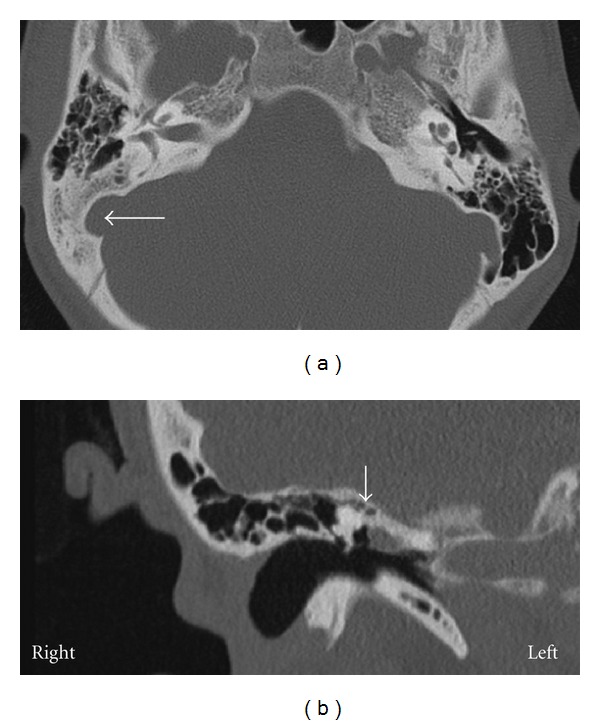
Computed tomography of the temporal bone. (a) Coronal section: lowered pneumatization of the mastoid (long horizontal arrow). (b) frontal section: narrow epitympanum with fixation of the ossicular chain (short vertical arrow).
